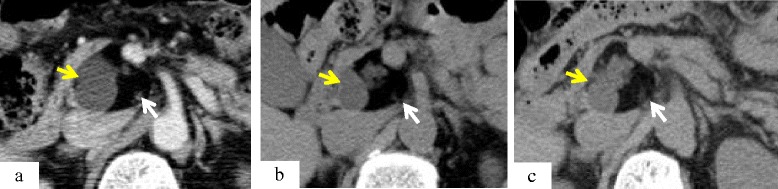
Chronological changes seen on CT. a: First examination. The hypo-enhanced mass was 4.2 × 3.9 cm in size with solid and cystic lesions located in the uncus of the pancreas. b: At 21 months after first examination. The tumor shown is 3.9 × 3.6 cm in size. The cystic lesion (*yellow arrow*) had become smaller and the solid lesion (*white arrow*) had become larger. c: At 28 months after first examination. The tumor was 4.2 × 3.3 cm in size. The cystic lesion changed and displayed irregular margins
